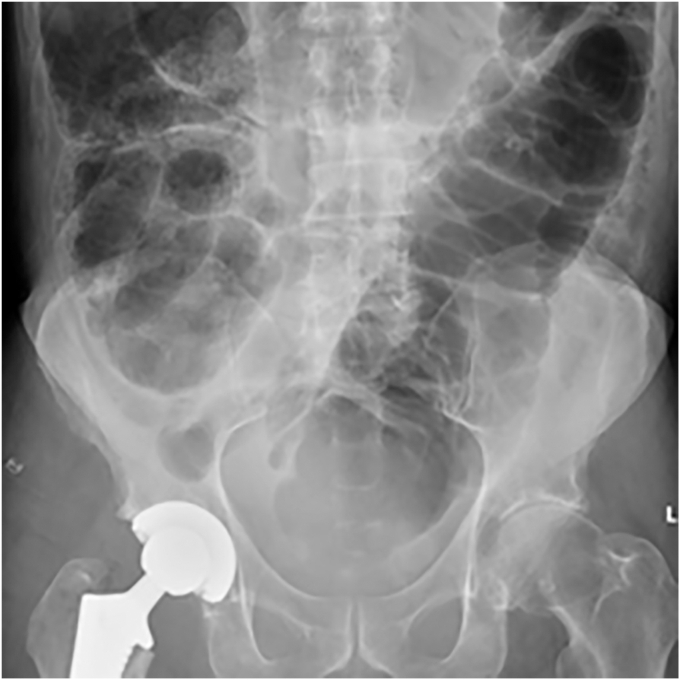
Abdominal film showing right-sided colon distension with air in the rectum.•CT of the abdomen and pelvis reveals proximal colonic dilation that may extend to the rectum while ruling out colonic obstruction. When a CT is not available, a contrast enema using water-soluble contrast can be used, providing there is no evidence of perforation on examination.^1^
